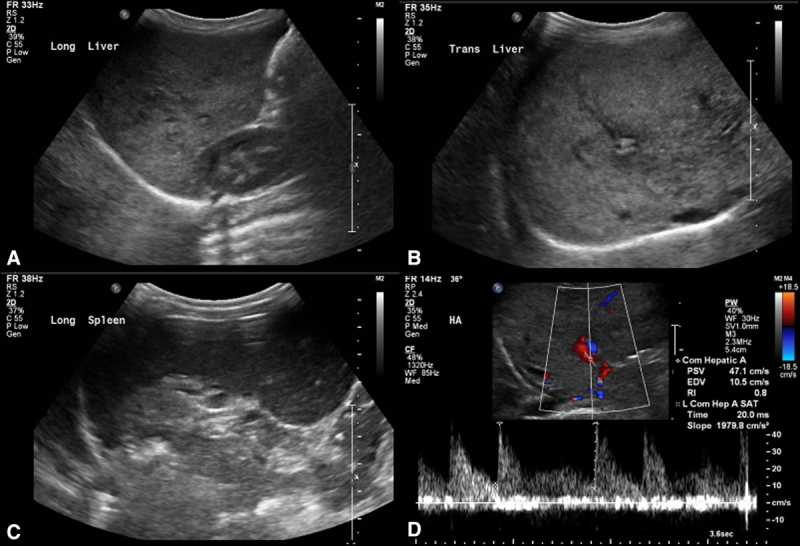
Ultrasound liver and spleen findings. Liver parenchymal echotexture is heterogeneous and hyperechoic (A and B). Spleen is enlarged (C). Hepatic artery demonstrates elevated resistive index at 0.8 (D).
